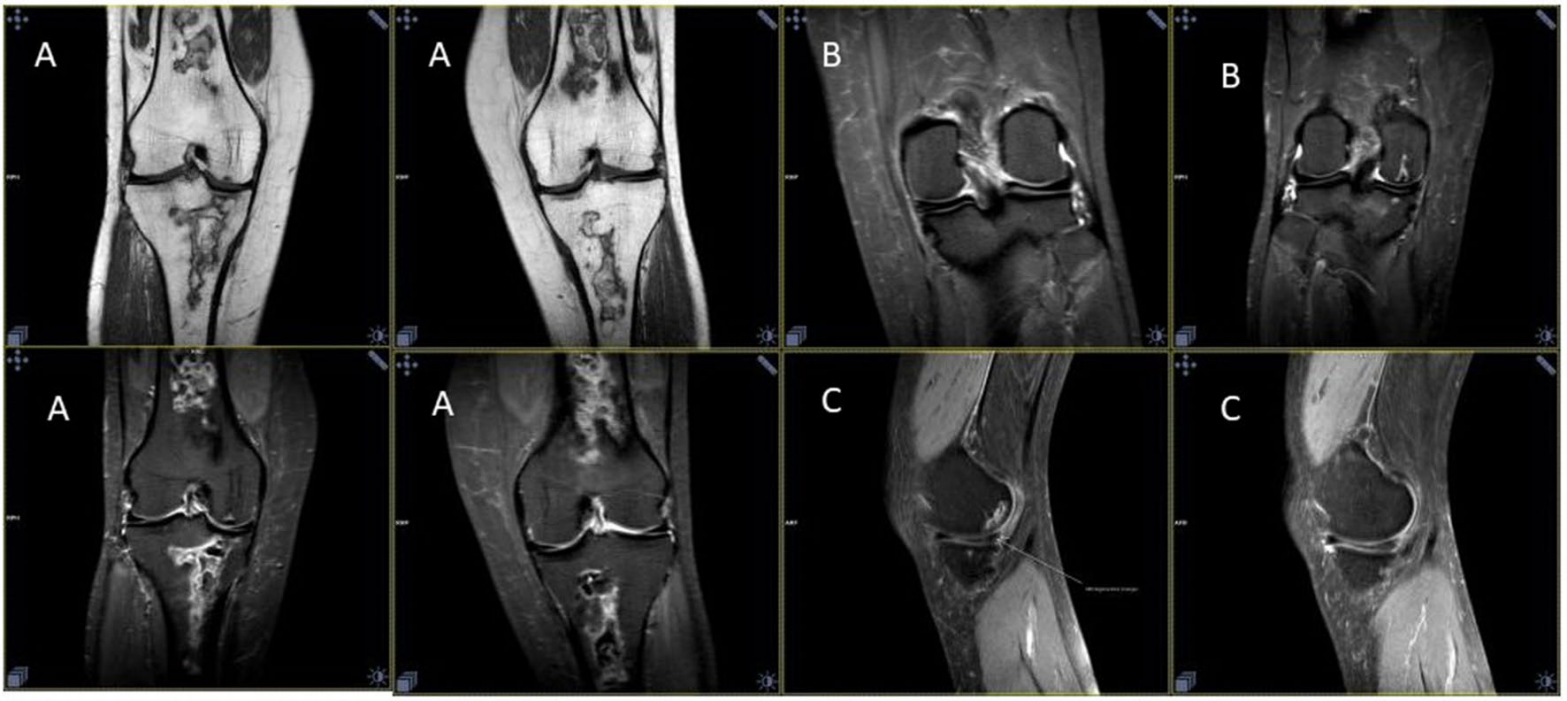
MRI of the knees. A Bilateral extensive regions of bone infarction in the shaft, epiphysis and metaphysis of femur and tibia. B Bilaterally 2nd grade chondropathy in the zones of femur condyle support. C Degenerative changes of medial meniscuses in the body and posterior horn as well as 2nd grade chondromalacia of the patella
